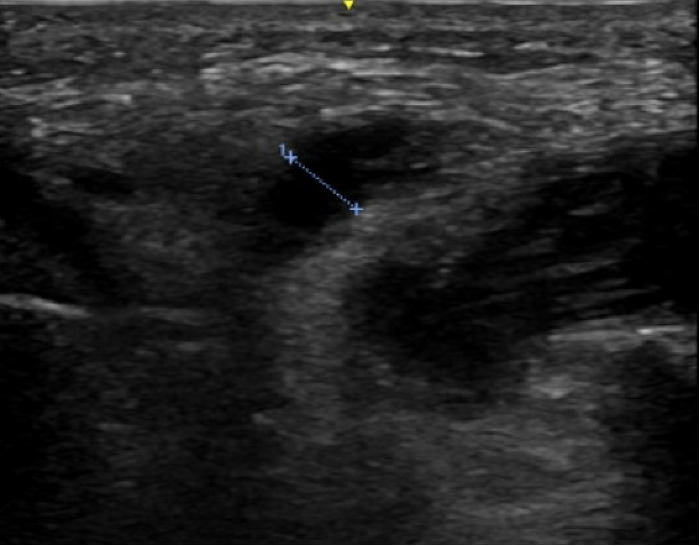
Left parotid duct (4.6 mm), transverse view using point of care ultrasound (POCUS).
